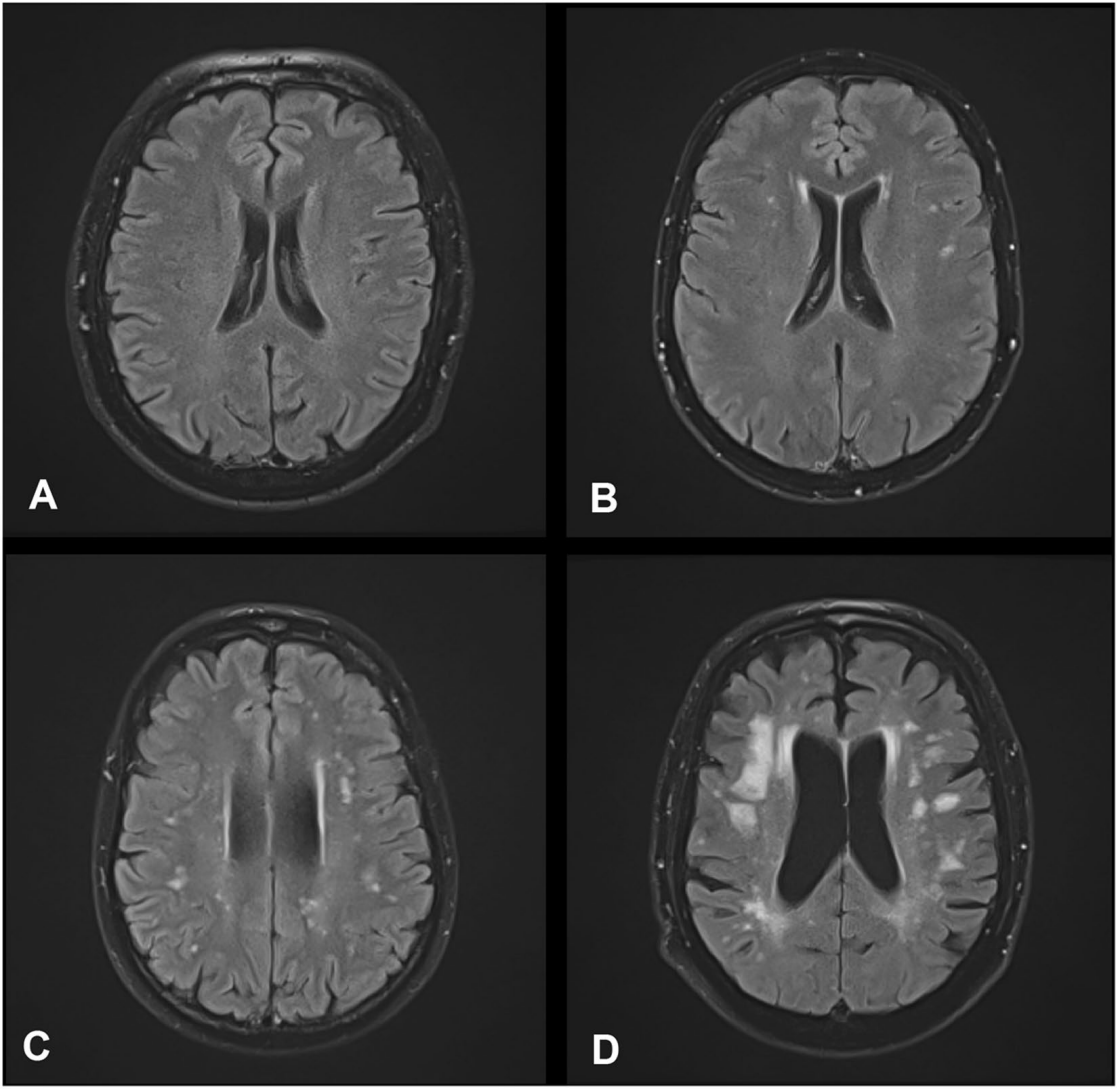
Example of the applied Fazekas scoring system (FS) for quantification of deep white matter hyperintensities (dWMH) on axial fluid-attenuated inversion recovery MRI (FLAIR). (A) Absence of hyperintense lesions in the deep white matter on FLAIR MRI (FS 0). (B) Punctate hyperintense foci of dWMHs on FLAIR MRI (FS 1). (C) Beginning confluence of dWMHs on FLAIR MRI (FS 2). (D) Large confluent areas of hyperintense lesions on FLAIR MRI (FS 3).
